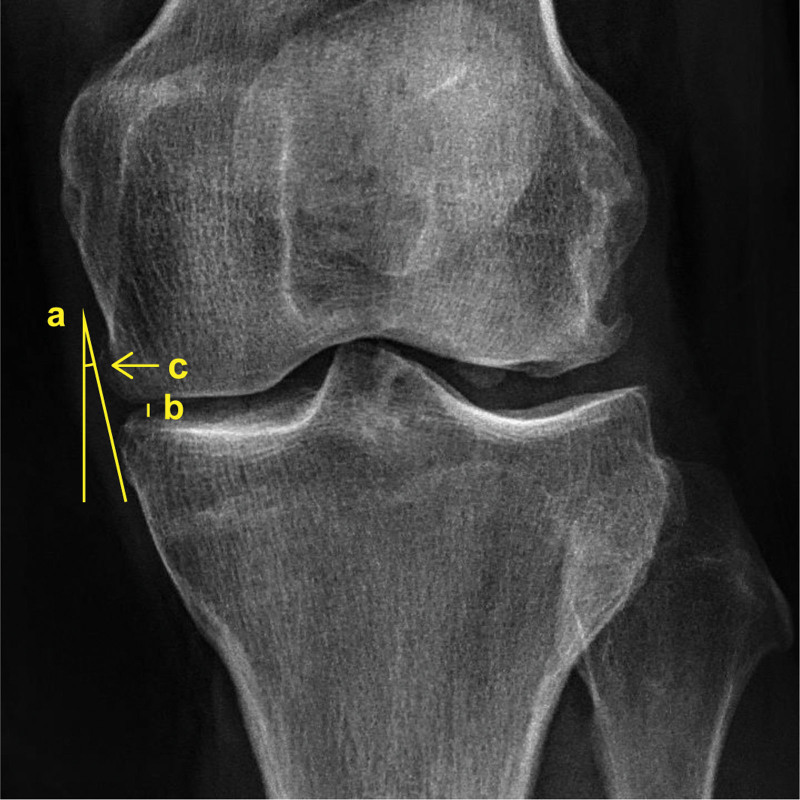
Preoperative anteroposterior knee radiograph. a, medial edge incline angle: the angle between the common tangent to the medial edges of the femur and tibia and the vertical line; b, medial joint space: the length of the vertical line segment from the medial edge of the tibia plateau to the femoral condyle; c, osteophyte on the medial edge of the femoral condyle.
